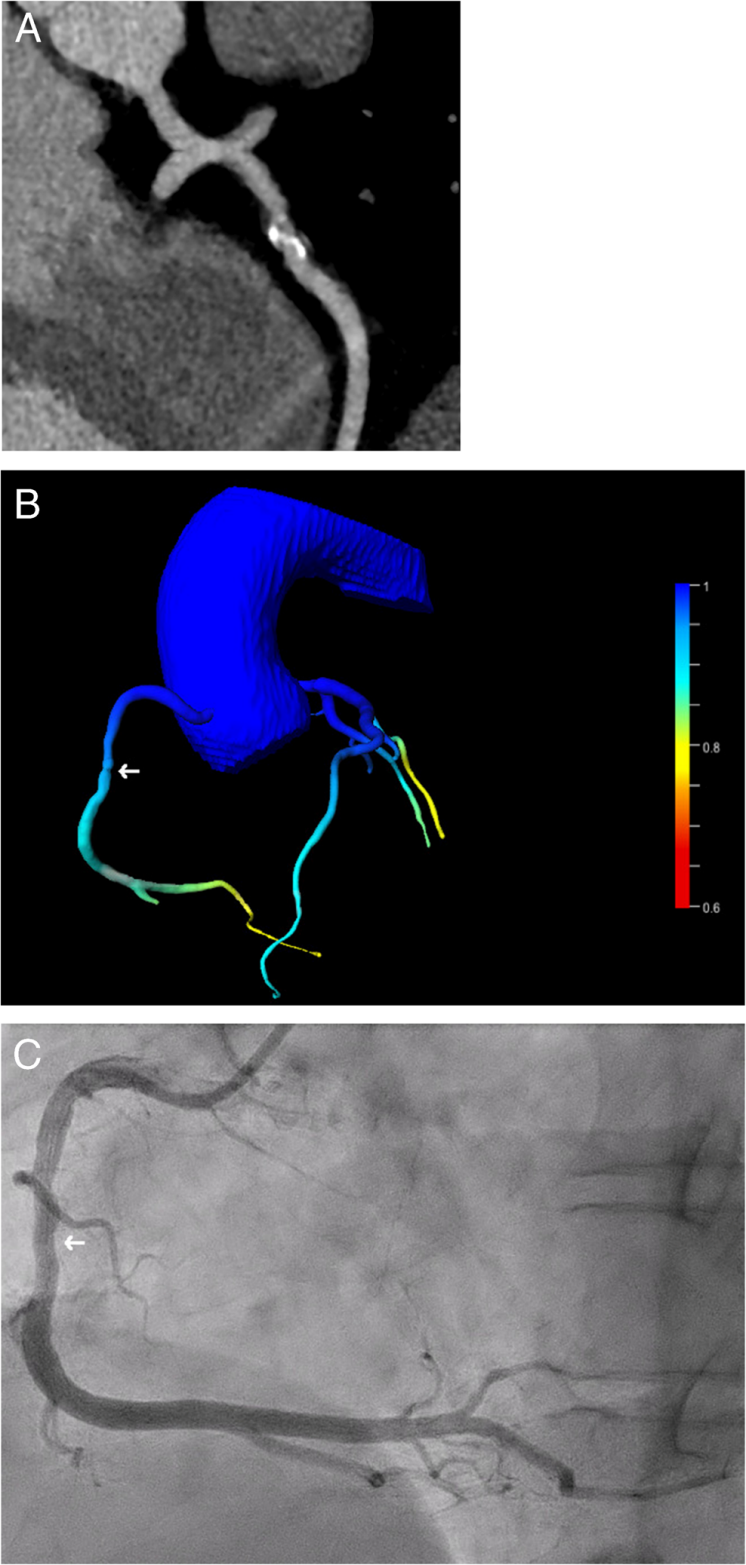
A 50-year-old male patient undergoing evaluation for liver transplantation. (A) Curved multiplanar reconstruction of the CCTA: A significant stenosis of the RCA was suspected (vessel lumen pre-stenosis 10.4 mm^2^, in-stenosis 4.5 mm^2^, post-stenosis 10 mm^2^); (B) Calculation of CT-FFR revealed hemodynamically non-significant stenosis (→, CT-FFR 0.87); (C) This finding was confirmed in ICA (→, iFR 0.97)
